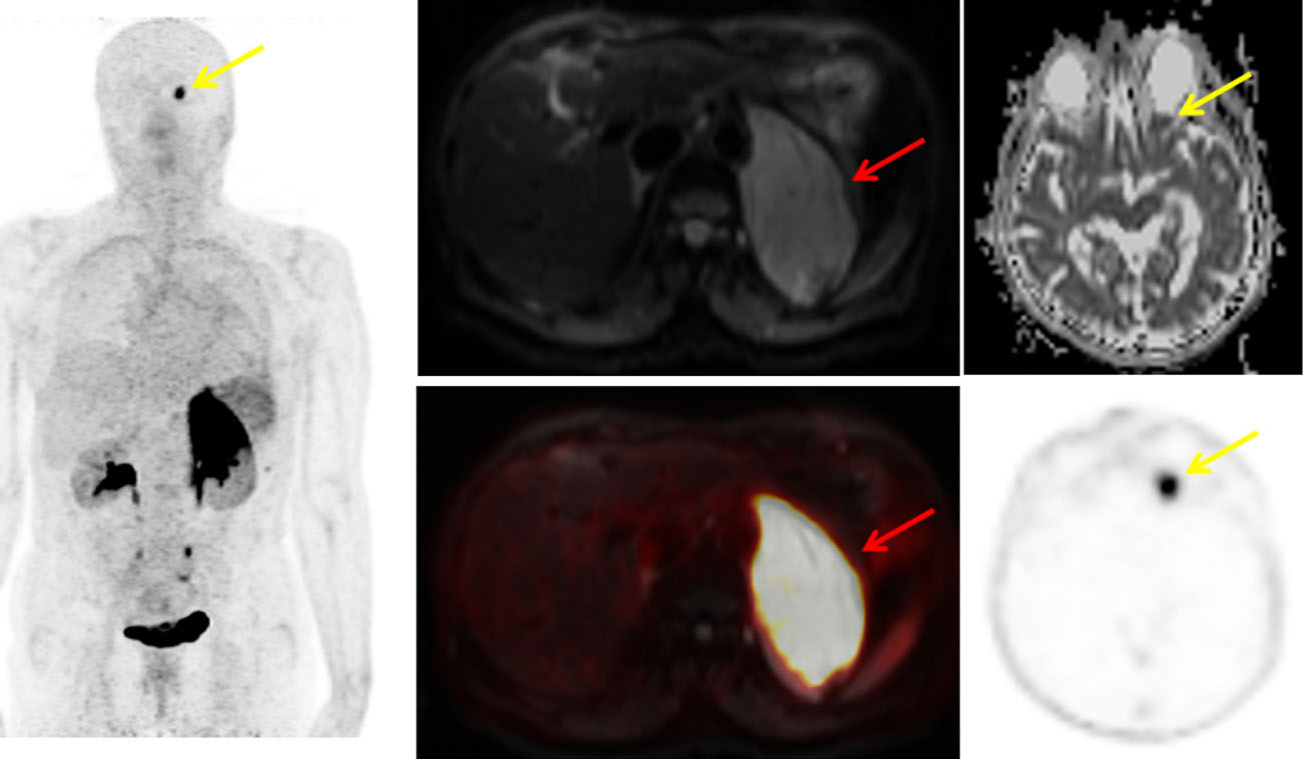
^68^Ga-Pentixafor PET in MALT lymphoma. A 75-year-old woman with biopsy-proven MALT lymphoma of the pararenal region. The known large MALT lymphoma shows high ^68^Ga-pentixafor uptake (SUVmax 25.4) and restricted diffusion on the diffusion-weighted images with b value 800 (*red arrows*). In addition an area of increased uptake on the PET images with restricted diffusion on the ADC map (*yellow arrows*) is evident, which proved to be MALT lymphoma of the orbit
